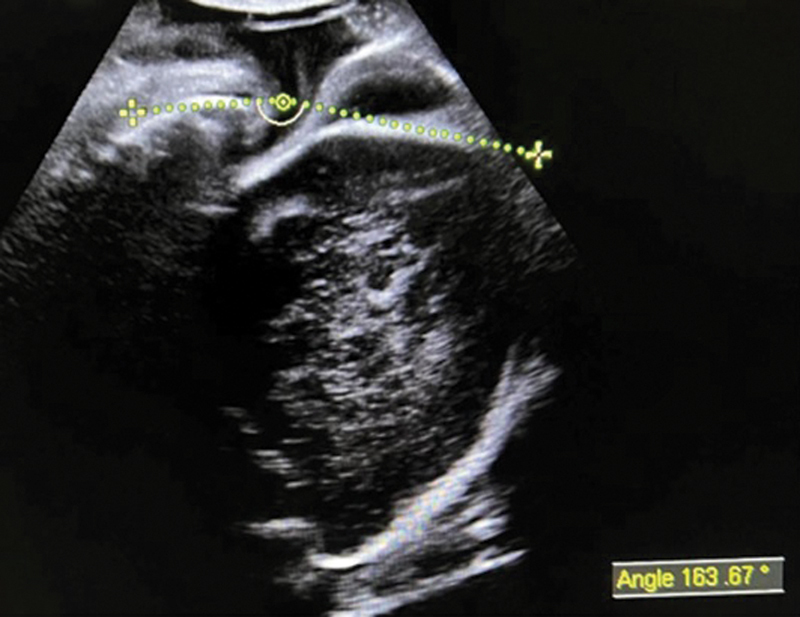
Ultrasound imaging demonstrating the angle of progression (AoP) access.
